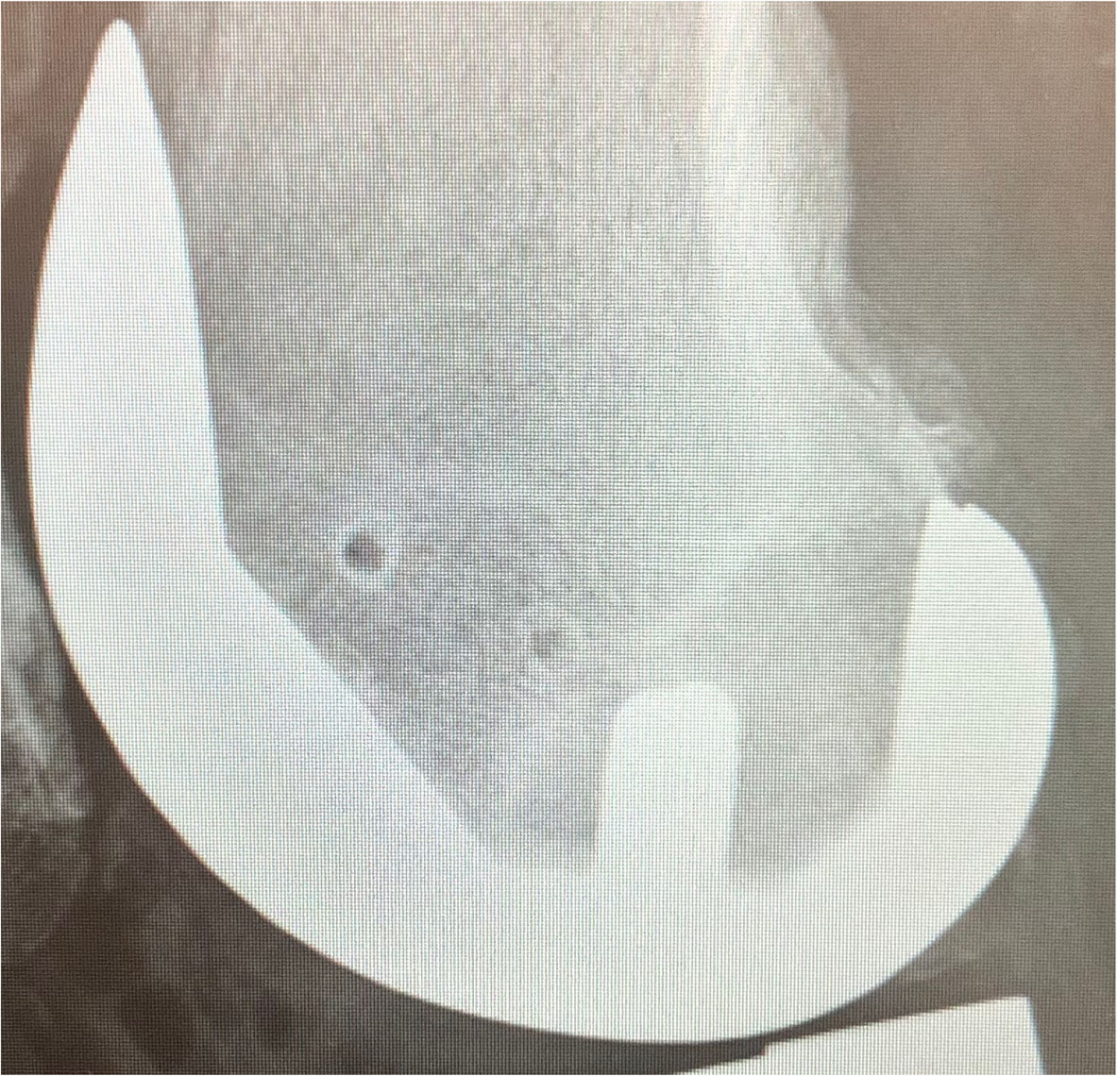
A lateral X-ray of TKA shows the position of the pin hole after periarticular femoral pin placement
